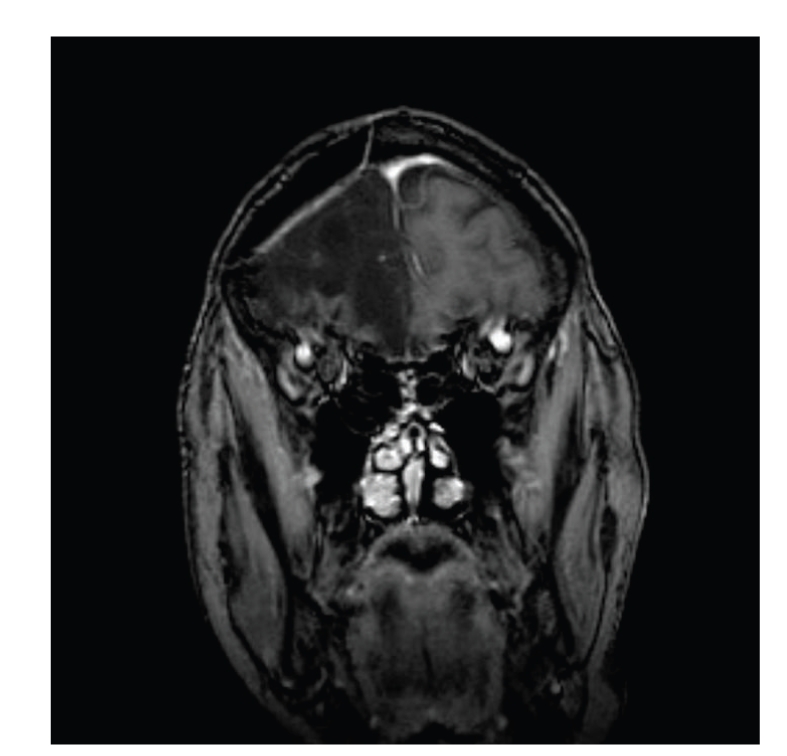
MRI of the brain showing no mass or enhancing lesion.
